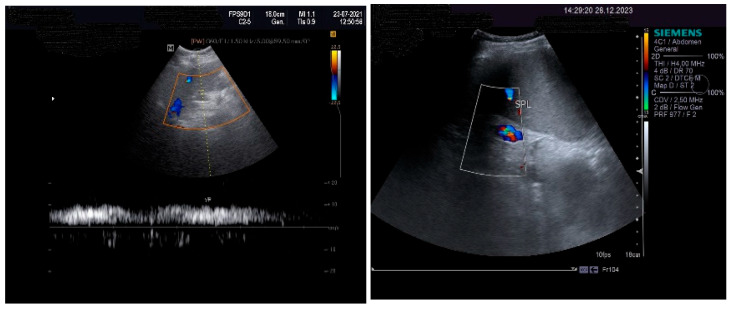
Doppler examination. Liver: pulsed Doppler of the portal vein (left). Spleen: color Doppler of splenic vein (right).
